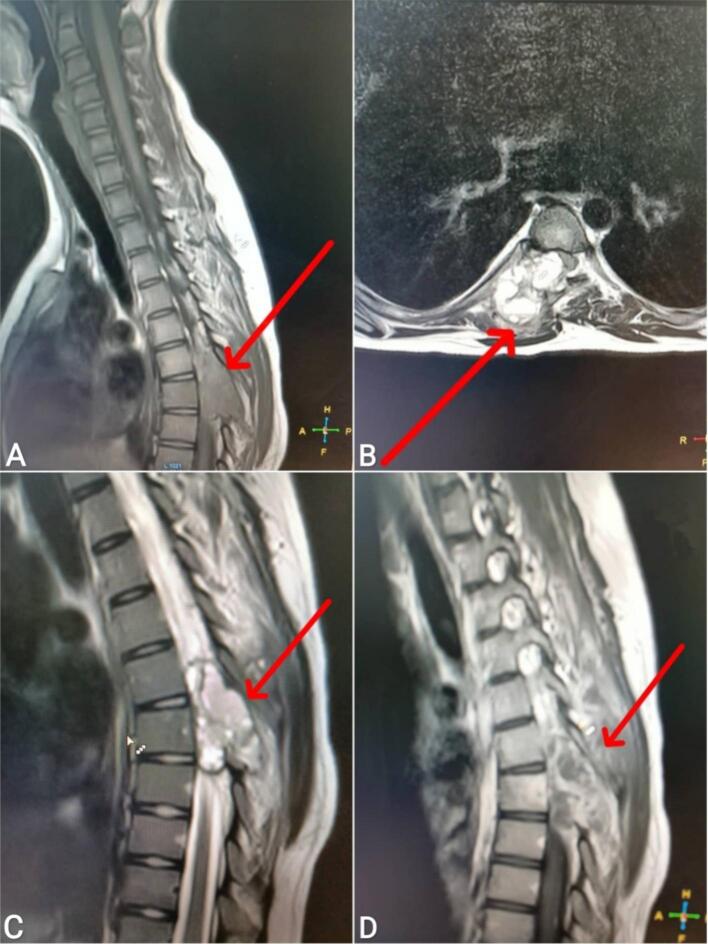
Non-contrast (images A, B, and C) and contrast (image D) magnetic resonance imaging revealed a tumorous lesion at the level of the D6 and D7 vertebrae, measuring about 4 cm × 4 cm × 5 cm. The lesion showed high signal intensity on T2-weighted images, low signal intensity on T1-weighted images, and heterogeneous enhancement post-contrast image, with erotion of the D6 posterior arch and the right pedicle, causing spinal cord compression. (A: Sagittal section of T1-weighted sequence, B: Axial section of T2-weighted sequence, C: Sagittal section of T2-weighted sequence, D: Sagittal section of T1-weighted sequence).
